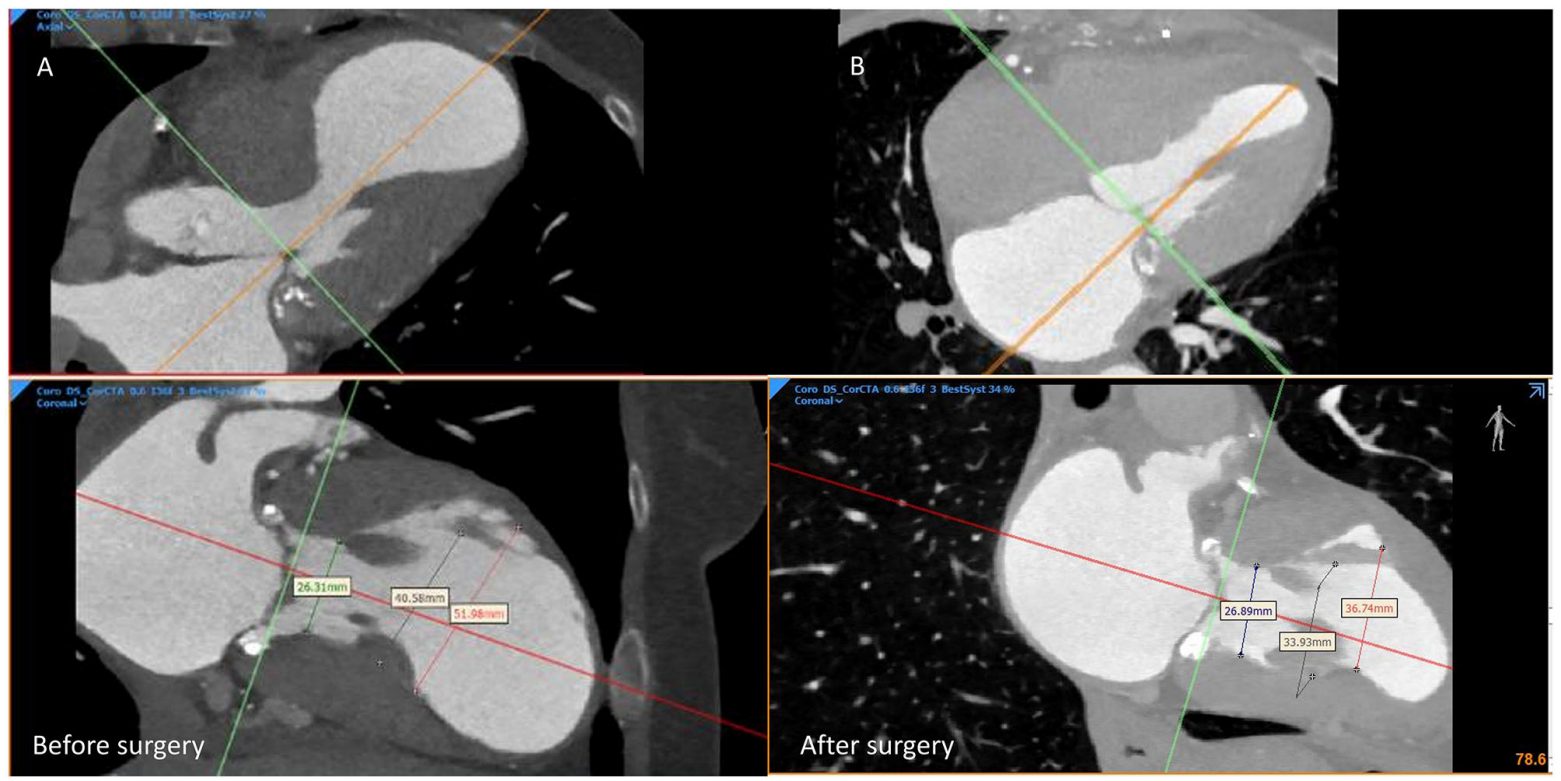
CT scan: the geometrical shape of the mitral valve, annulus, and papillary muscle attachment (A) before surgery, (B) after surgery.
